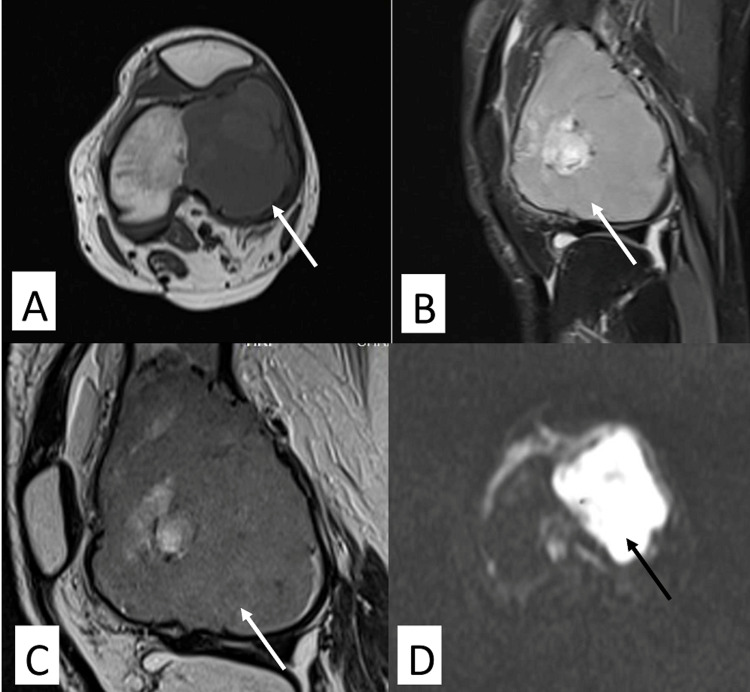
MRI images of the left knee joint showing lesion arising from the lateral femoral condyle (A) T1 axial section - lobulated hypointense lesion of the lateral femoral condyle (white arrow). (B) T2 sagittal section showing heterointense lobulated lesion (white arrow). (C) T2 thin sagittal section showing heterointense lobulated lesion (white arrow). (D) DIffusion weighted image showing diffusion restriction in the lateral femoral condyle (black arrow).
